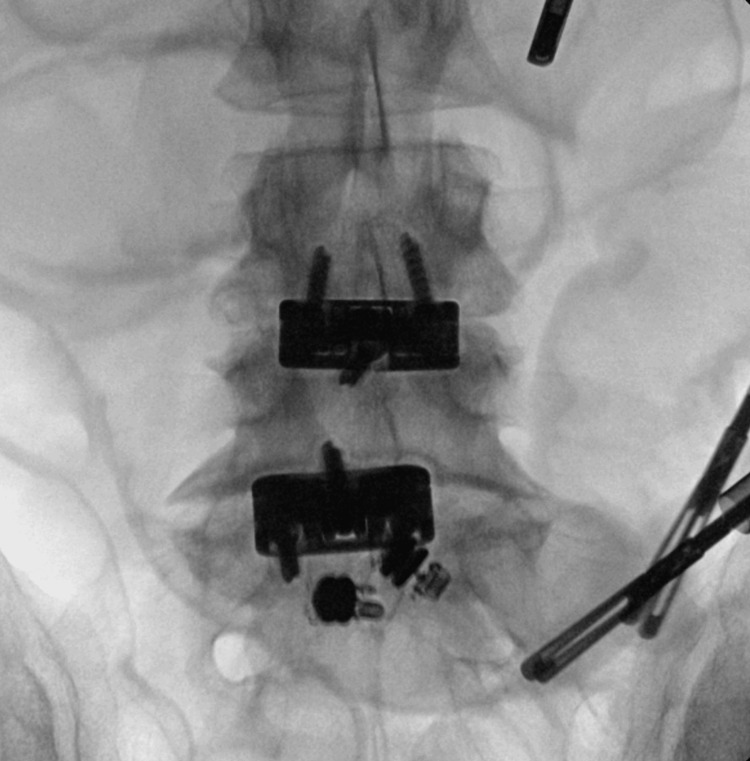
Intraoperative X-ray of video capsules before resection.
